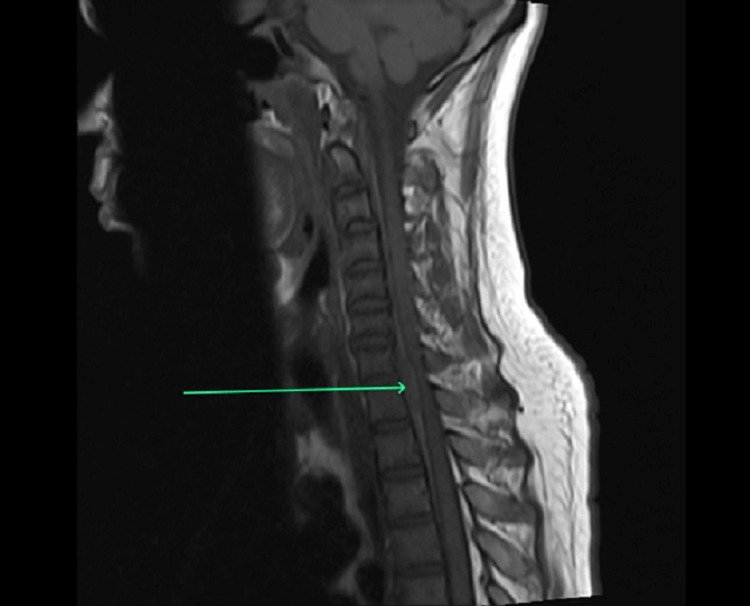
T1-weighted image of the sagittal section of the cervical spinal cord showing a well-defined extradural extramedullary isointense lesion along the anterior surface of the spinal cord extending from mid-C7 to mid-T1 vertebral levels (arrow).
